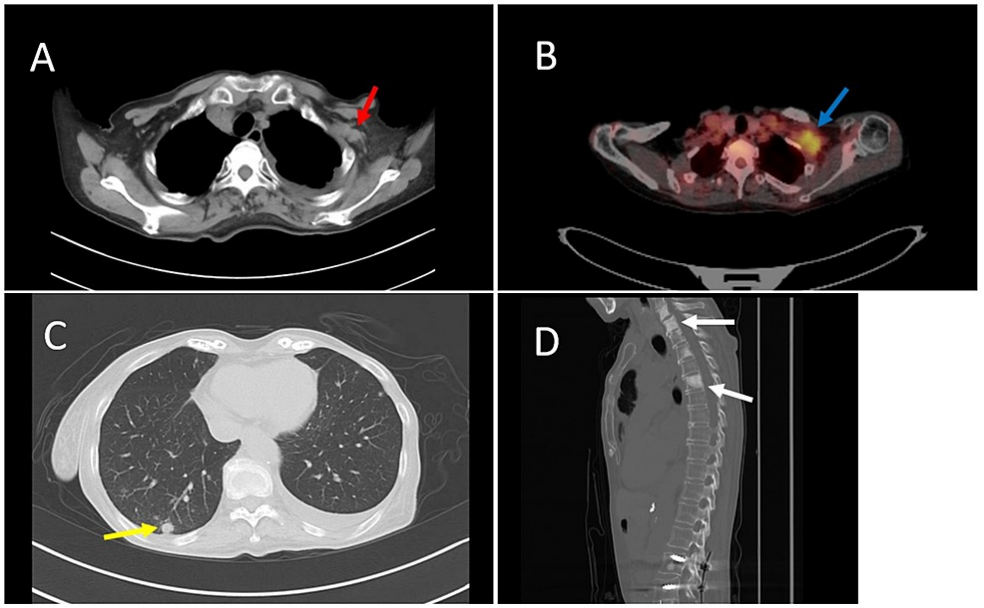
CT and PET-CT. (A, B) The red and blue arrows indicate lymph node metastases. (C) The yellow arrow indicates lung metastasis. (D) The white arrows indicate multiple bone metastases. CT: computed tomography; PET-CT: positron emission tomography-computed tomography
